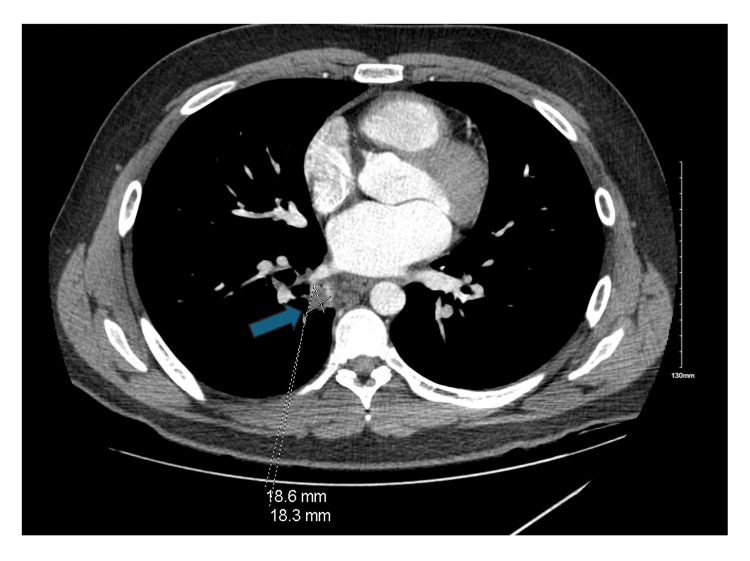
CT thorax three months later CT thorax with contrast three months after initial imaging demonstrating a significant decrease in the size of the cavitary lesion (1.8 cm) in the medial aspect of the right lower lobe.
